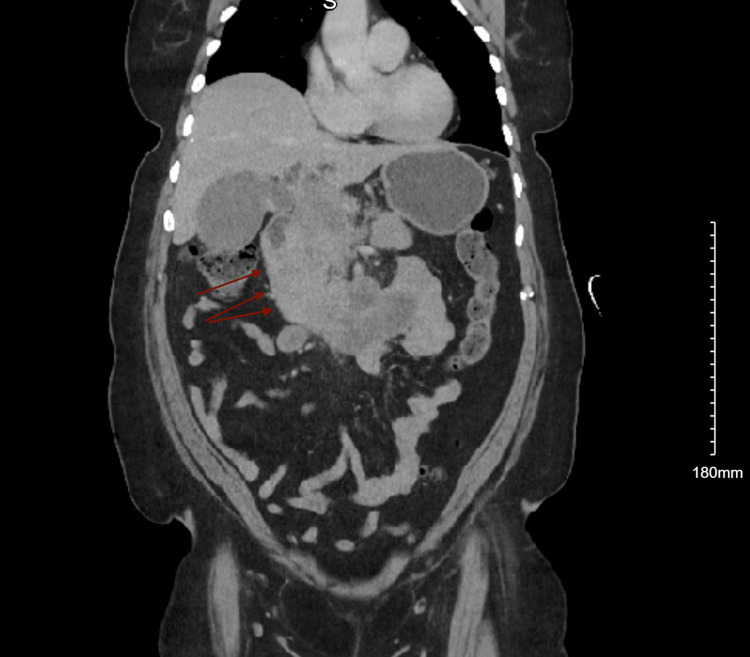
CT of the abdomen. Red arrows: non-discernible mass-like irregularity in the region of the pancreatic head.
